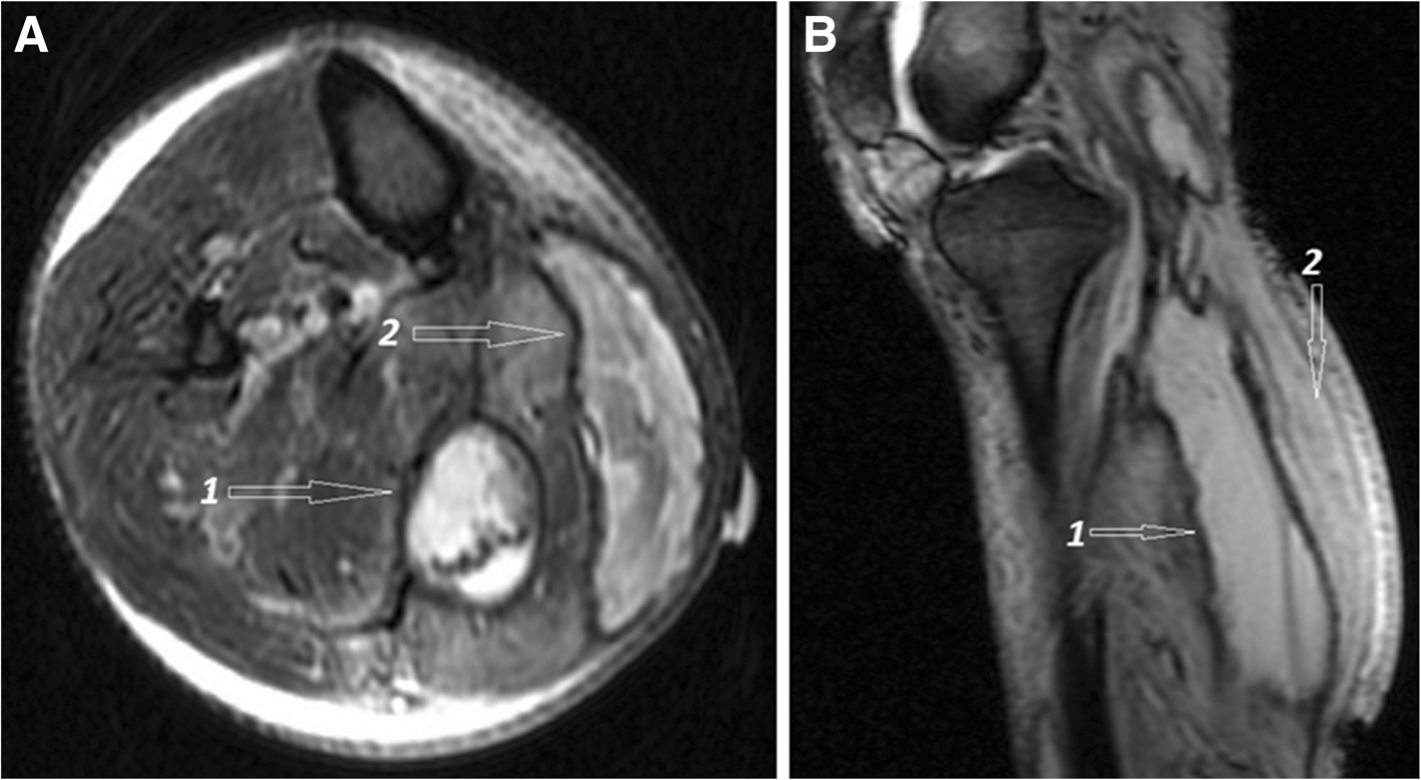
Selected axial (A) and sagittal (B) magnetic resonance images demonstrating two longitudinal hyper intense collections (1 and 2) within the medial gastrocnemius muscle. The hyper intense signal of the non-necrotic portions in the gastrocnemius and soleus muscles is indicative of inflammatory changes and edema.
